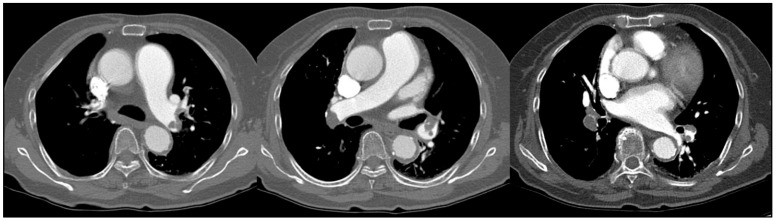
CT pulmonary angiography shows multiple PEs. Locations of PEs correspond to areas of dark blue areas on color overlayed ED images in Figure 3. PE, pulmonary embolism; ED, electron density.
